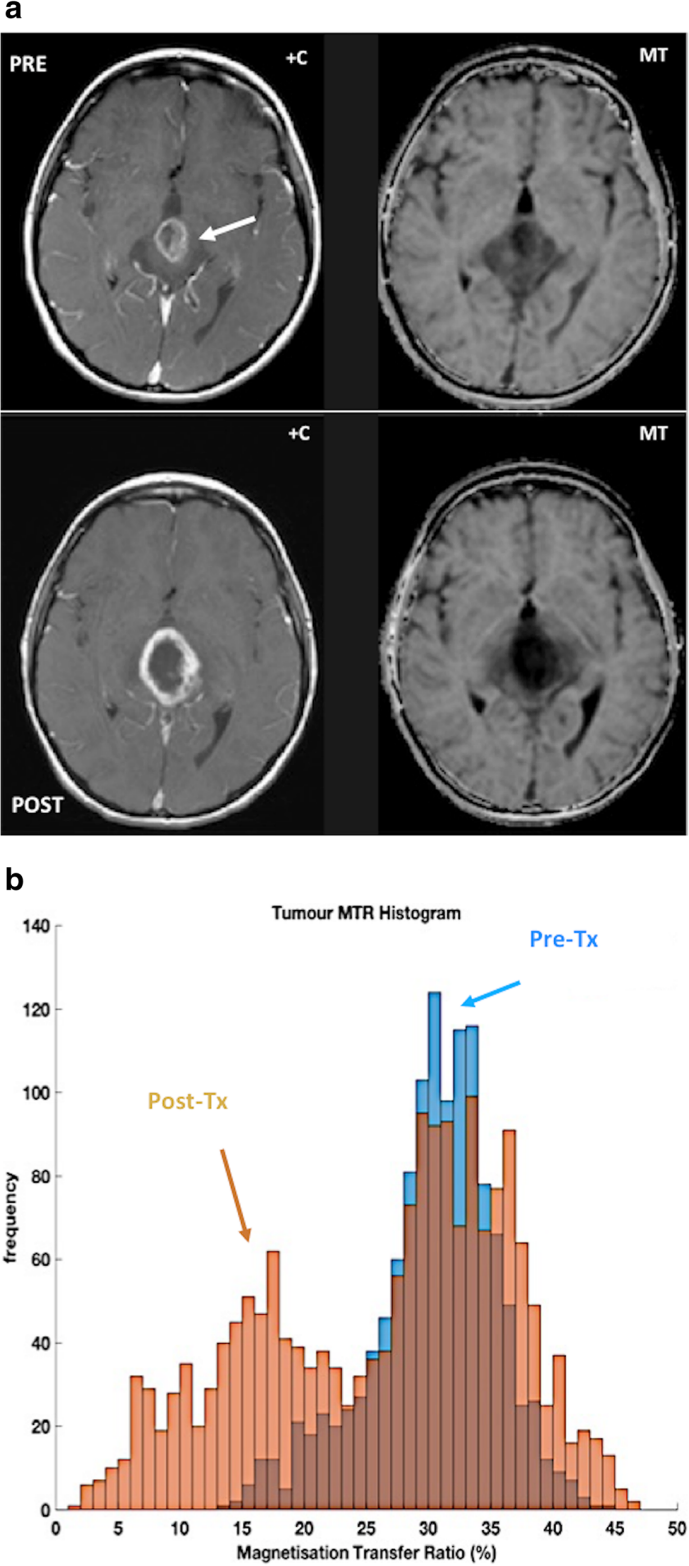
An 8-year-old patient with high-grade tectal plate glioma (white arrow). a Post-contrast T1-weighted (+C) and magnetization transfer (MT) images were obtained before (top row) and after (bottom row) chemoradiotherapy; and (b) the corresponding MT ratio histograms of lesion are as shown. The magnetization transfer acquisition comprised a set of four geometry-matched 3D-GRE scans: two flip angles (4 and 24°), with/without MT pulse (1.5 kHz offset) on a 1.5 T MR system. Note the reduction in the MT ratio (histogram in b) associated with response to treatment, accompanied by slight increase in size and enhancement of the tumor (a). [Images courtesy of Dr. Neil P. Jerome, Norwegian University of Science and Technology]
